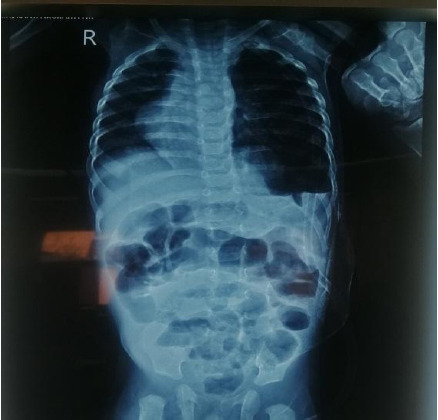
Post-operative chest X-ray showing lowered left hemidiaphragm with chest tube in-situ on the left side.
